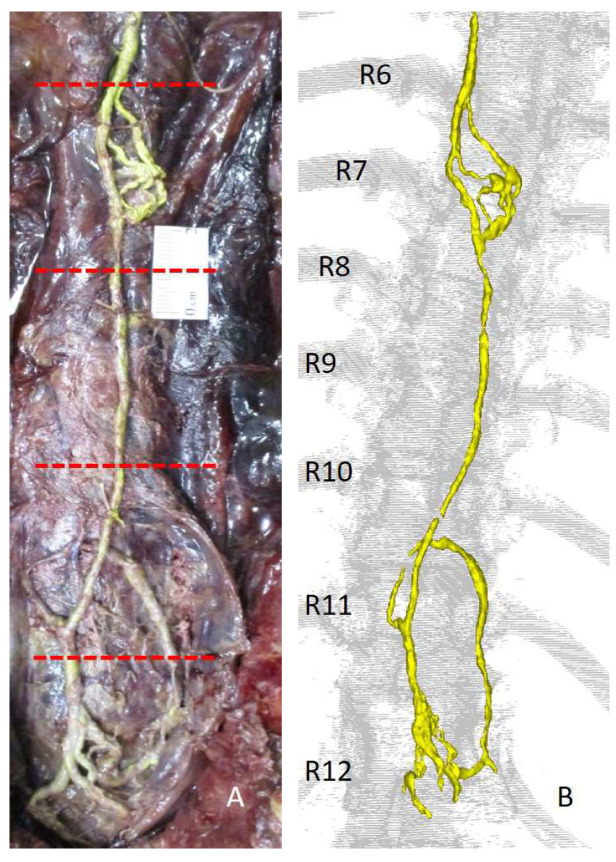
Dissection picture (A) and digital reconstruction from the corresponding CT scan (B), showing the contrast-filled lymphatics obtained after retrograde lymphatic injection of the thoracic duct. The red dotted lines in figure (A) show the possibility to make standardised test units to test retrograde lymphatic injection approaches by ligating the thoracic duct at equally spaced intervals (5 cm). The thoracic duct is shown in yellow. Note how the thoracic duct in the dissection picture and the 3D reconstruction perfectly resemble each other. Also note that this set up provides easy, unobstructed access to the thoracic duct, allowing easy cannulation and visual control of contrast agent advancement during injection. R6–R12 = rib 6–rib 12. Adapted from Stouthandel et al. [72].
